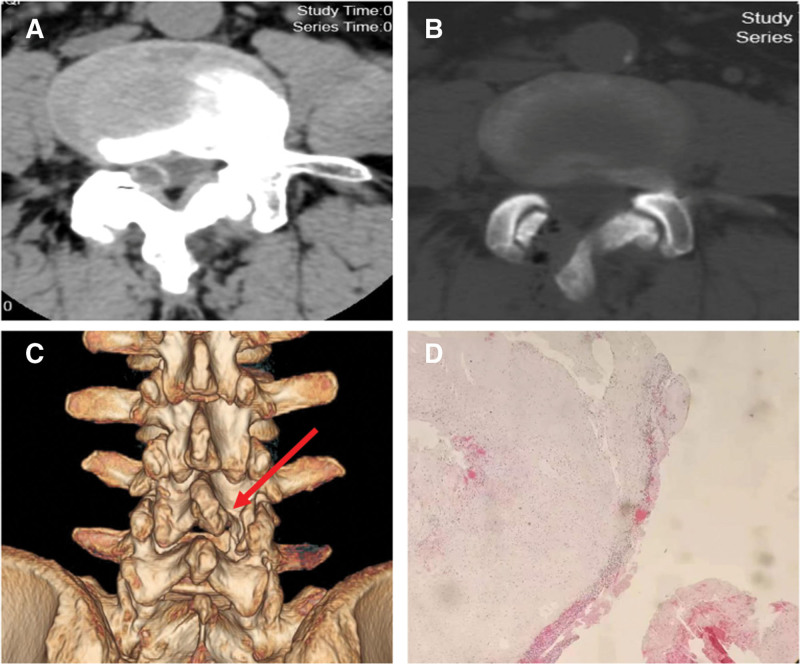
(A) Preoperative CT showed a round low-density shadow on the right side of the spinal canal at the L4/5 level. (B and C) Three months after the operation, lumbar spine CT and 3-dimensional reconstruction showed that the L4/5 level tumor was completely removed, with no recurrence, and the facet joints were well preserved. (D) The postoperative pathological examination results showed cystic wall-like changes in connective tissue without epithelial cell tissue. CT = computed tomography.
